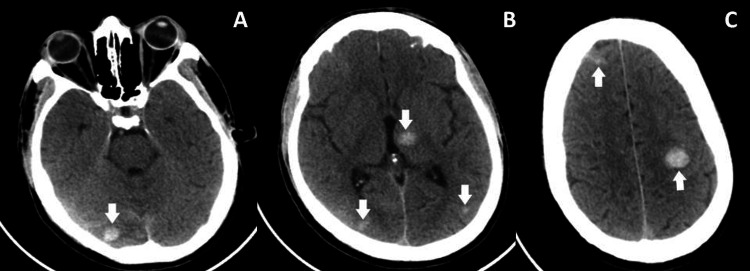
Axial cuts of cranial CT (in the caudocranial direction: A-C) revealing multiple foci of hemorrhagic transformation (arrows)
